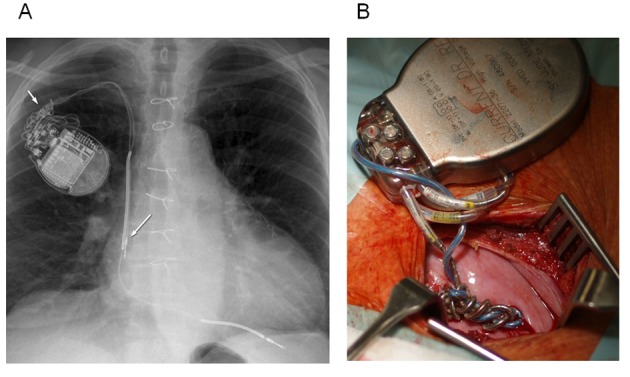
(A) The chest X-ray was indicative of Twiddler’s syndrome demonstrating retraction of the atrial lead and excessive coiling near the ICD header (see arrows). (B) Image taken during surgical revision of the ICD system showing coiled leads in situ still connected to the ICD.
